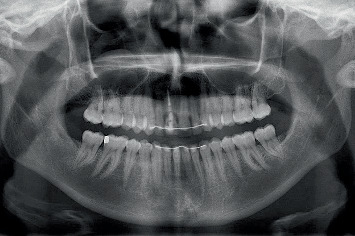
Radiographic view showing the failing maxillary right central incisor and donor site.
